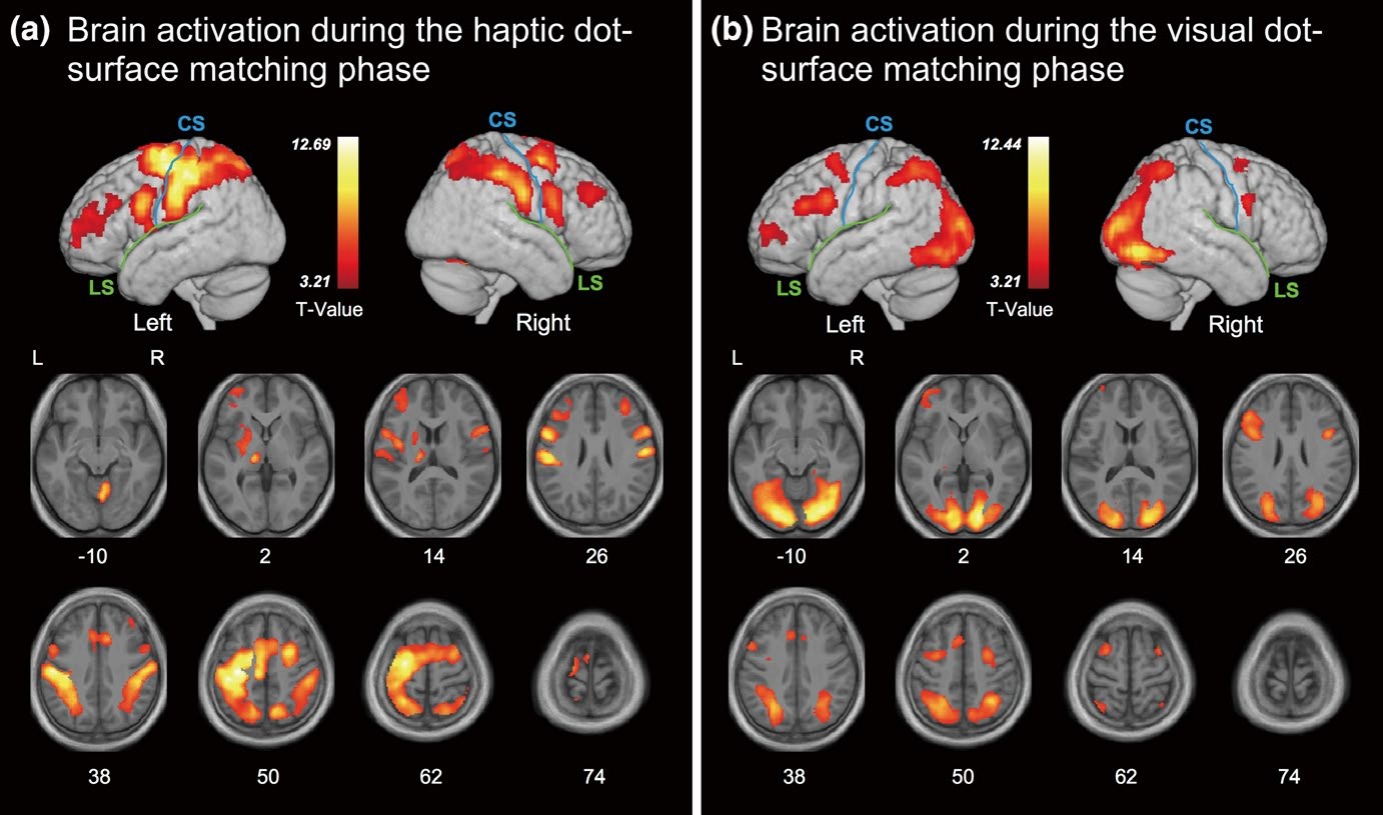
Brain activation patterns for haptic and visual dot‐surface match processing. (a) Common brain activation for the haptic dot‐surface‐matching phase. The results of the contrast (hH > rest) ∩ (vH > rest) are shown. (b) Common brain activation for the visual dot‐surface‐matching phase. The results of the contrast (vV > rest) ∩ (hV > rest) are shown. Areas of significant activation are rendered on a normalized T1‐weighted high‐resolution brain MRI averaged across 18 subjects and the axial section of the same image. The solid blue and green lines shown in the rendered images indicate the central sulcus (CS) and lateral sulcus (LS), respectively. The extent threshold for activation was *p* < .05, corrected for each search volume with a height threshold of *T* (68) = 3.21 (equivalent to *p* < .001, uncorrected)
